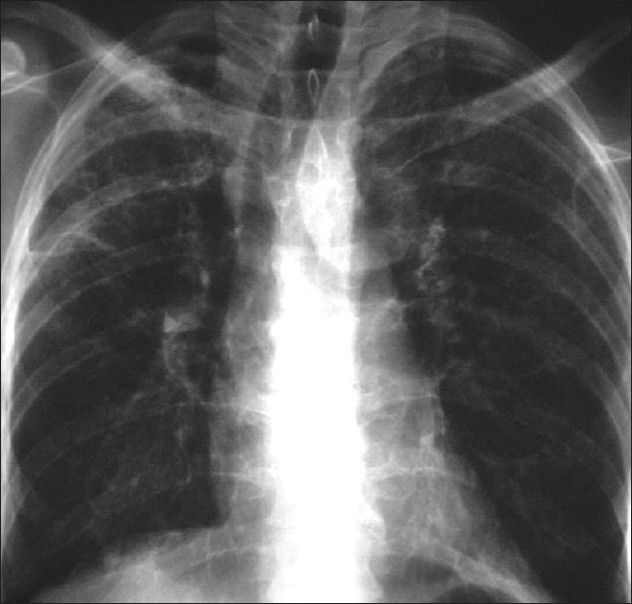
Pneumocystis carinii* pneumonia. Chest X-ray shows atypical features of upper lobe focal reticulation associated with minor ground-glass appearance
